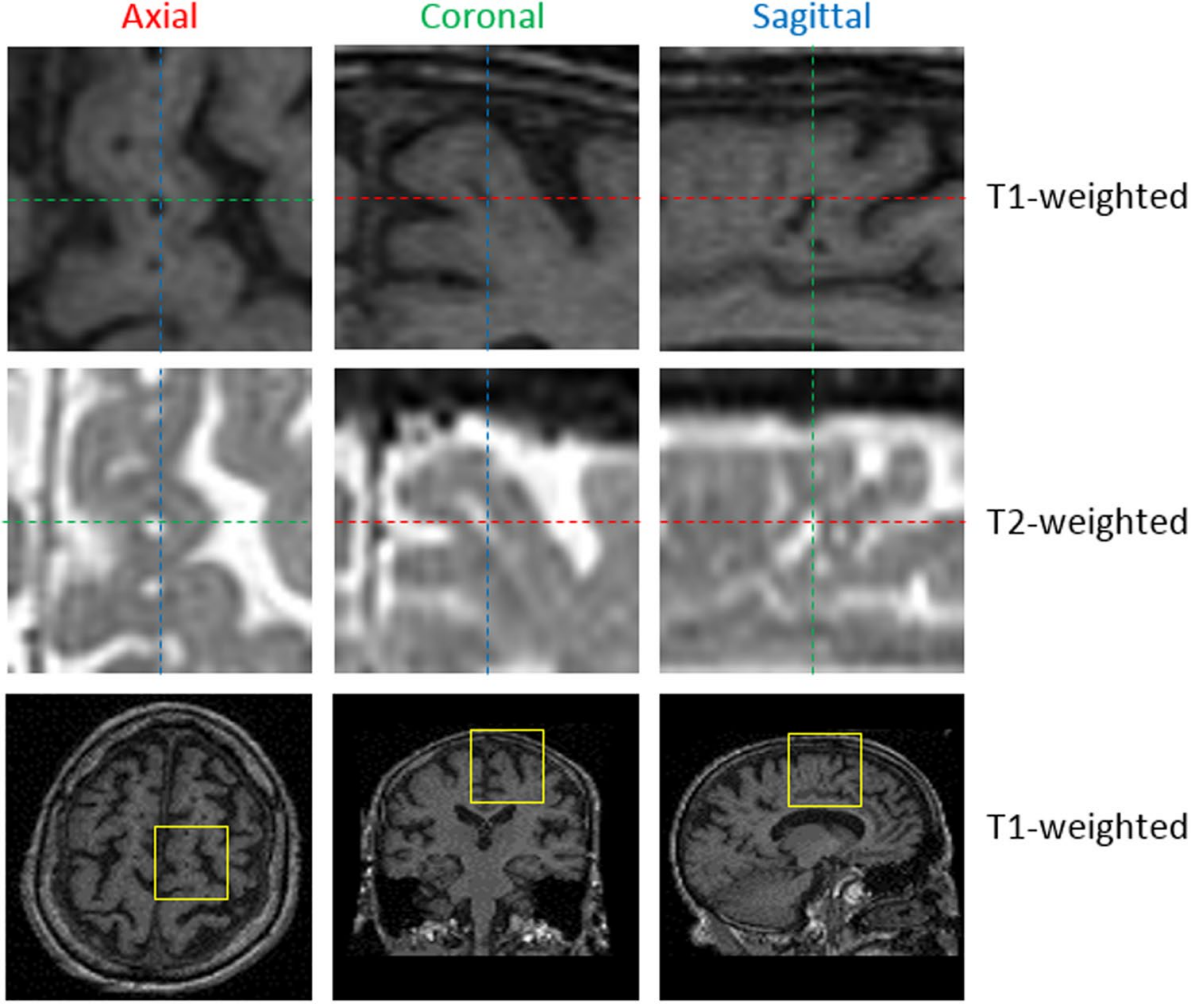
Magnified view of PVS in an axial, coronal and sagittal slice in T1-weighted and T2-weighted MR images. The position of these zooms in corresponding T1-weighted brain scans is highlighted with yellow squares (bottom row).
